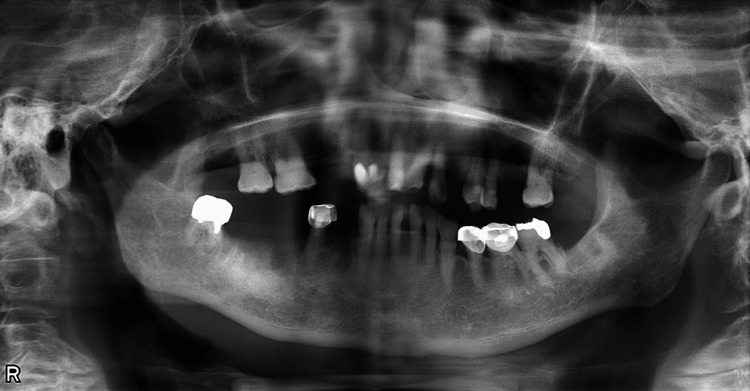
Post-treatment panoramic radiograph The oral focus of infection was successfully removed.
